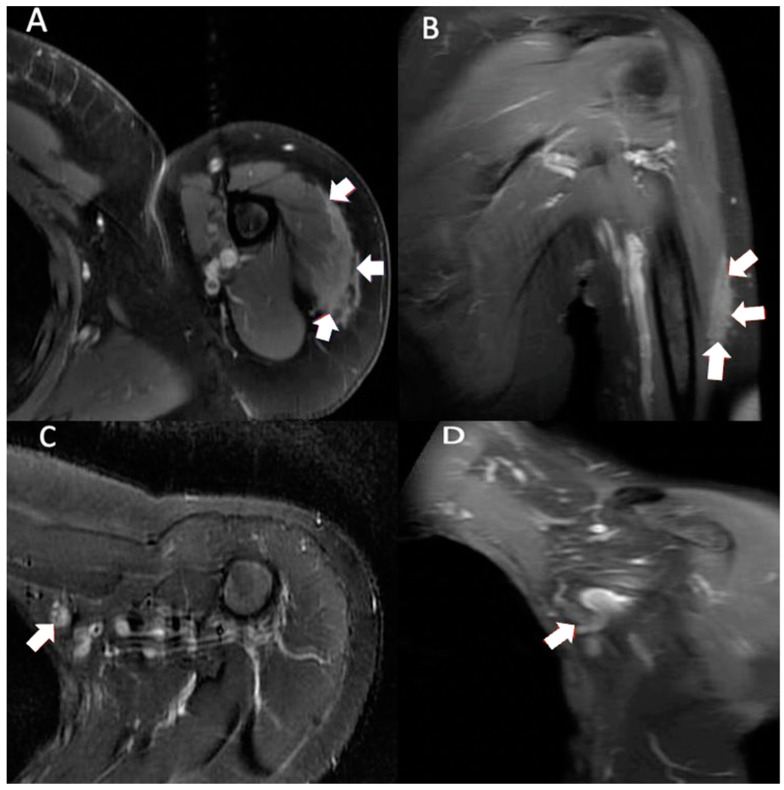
Multiplanar MRI of left shoulder taken 10 months after vaccine administration in left shoulder. Axial T1 weighted (A) image shows predominately intramuscular edema within the lateral deltoid muscle measuring 5.6 cm anterior-posterior and 1.0 cm medial-lateral (arrows). Coronal T1 weighted (B) image shows intramuscular edema within the lateral deltoid muscle measuring 10.0 cm proximal-distal (arrows). Axial T2 STIR (C) and coronal T1 weighted (D) images show a slightly enlarged left axillary node (9.9 × 11.2 × 12.8 mm) (arrows).
